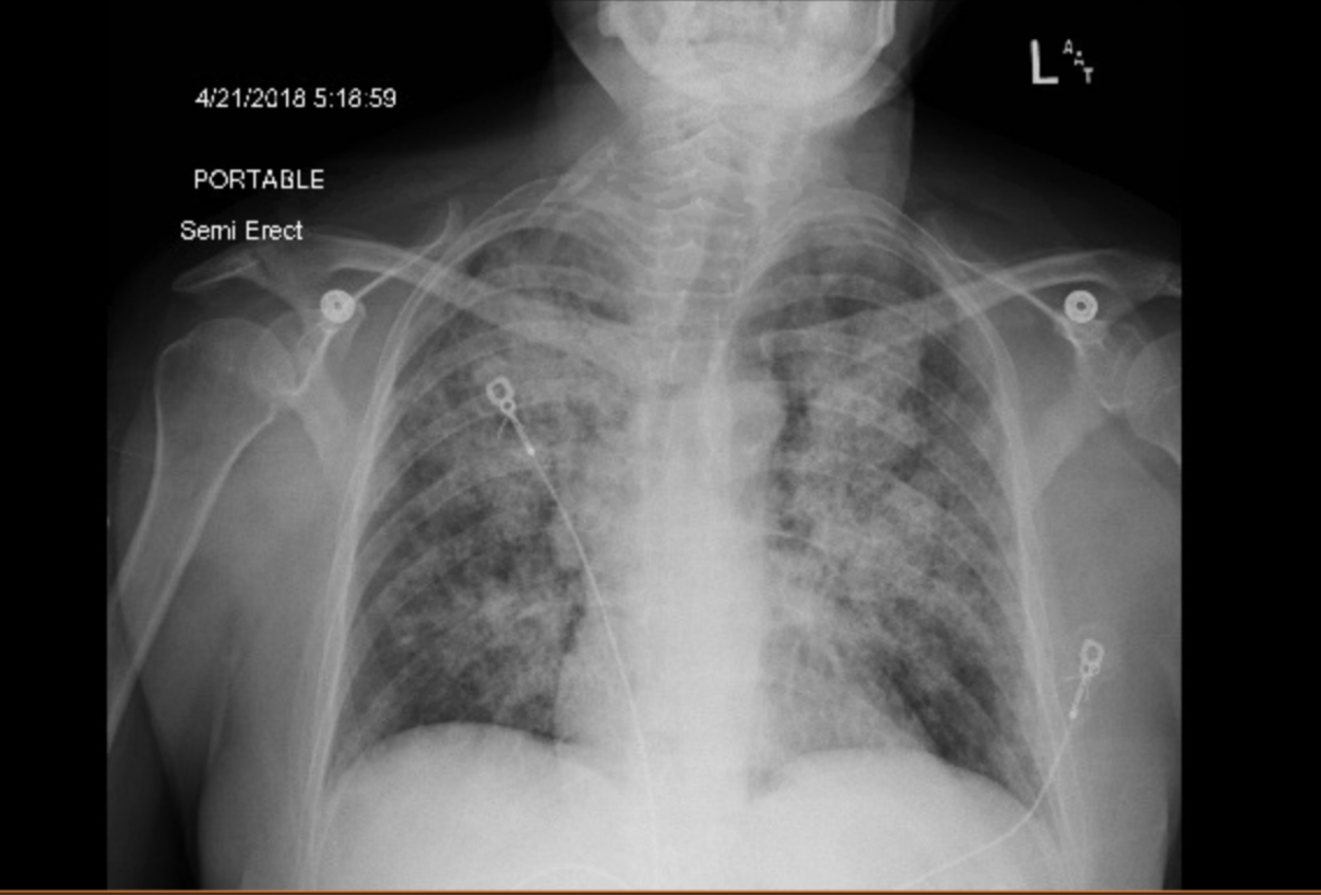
Repeat anterior-posterior chest X-ray obtained for increasing shortness of breath, showing diffuse multifocal airspace opacities consistent with acute respiratory distress syndrome
